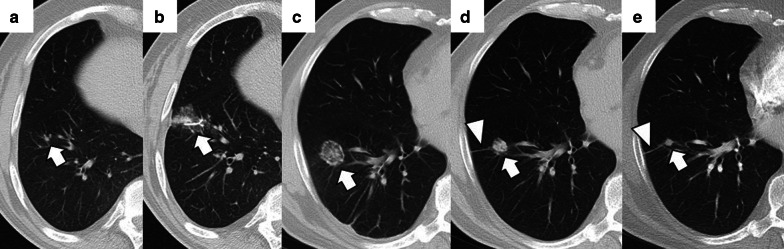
Evolution towards a nodule pattern after successful treatment with image-guided percutaneous lung ablation. Non-enhanced CT showing a small metastatic lung tumor from a rectal carcinoma in the lower right lobe (arrow in a). The CT scan performed 24 h after treatment with lung RFA shows a central consolidating area surrounded by a ground-glass opacity at the site of ablation (arrow in b). Although the ablative area is larger than the original tumor in the radiologic control performed one month after treatment, it has decreased in size compared to the post-ablative image (arrow in c). After six months of treatment, only a residual consolidation of rounded morphology remains in the treated area (arrow in d), with an adjacent lung scar (triangle in c, d)
